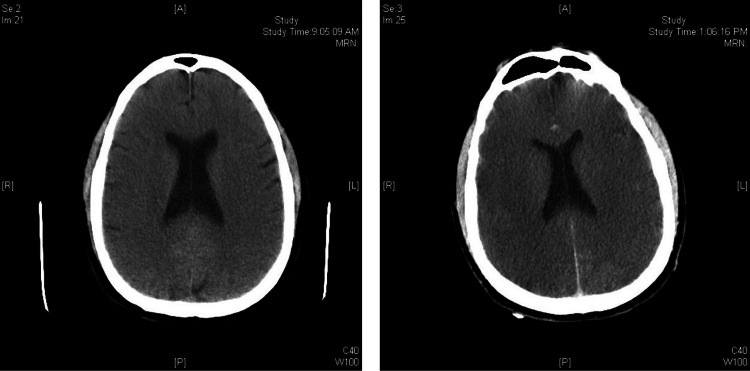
Brain CT showing interval worsening and bilateral cerebral edema, worrisome for hypoxic injury A: anterior, P: posterior, R: right, L: left.
